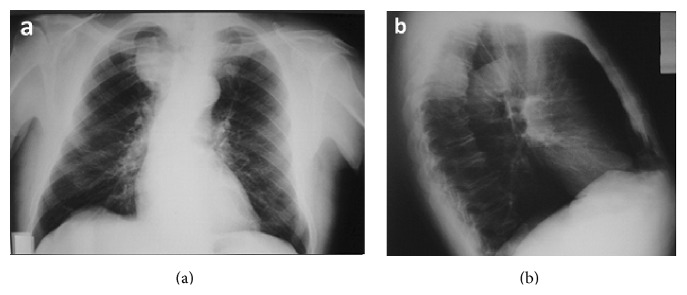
Chest X-ray film showing the tumor located to the posterior mediastinum.
